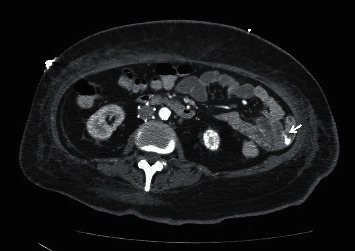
CT angiogram of the abdomen showed active bleeding in the jejunum (arrow).
